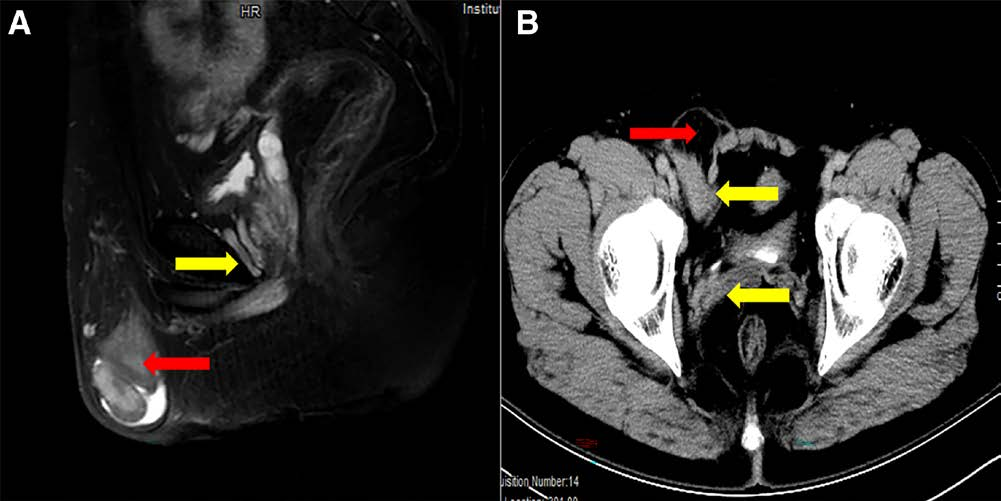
Preoperative MRI (A) and CT (B) images. Right hernia sac and tissue inside the hernia sac (red arrow). Abnormal “uterus” (yellow arrow). CT = computerized tomography, MRI = magnetic resonance imaging.
